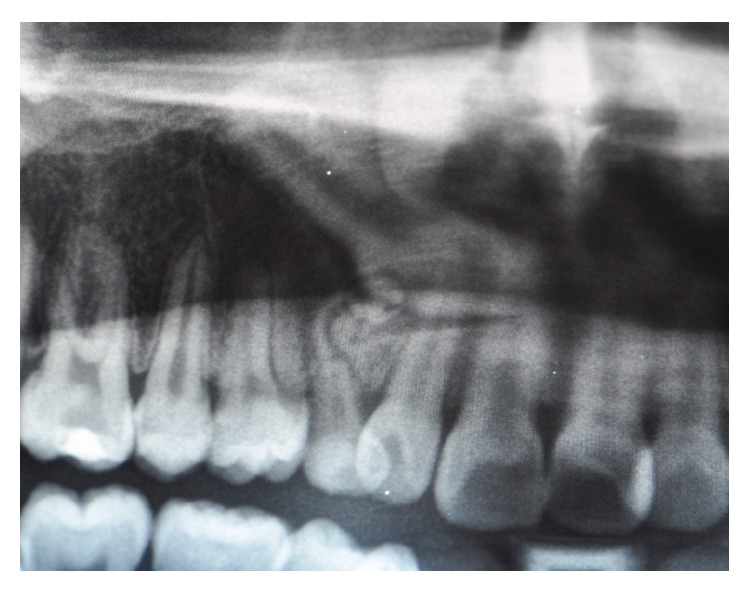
Panoramic X-ray (detail).
